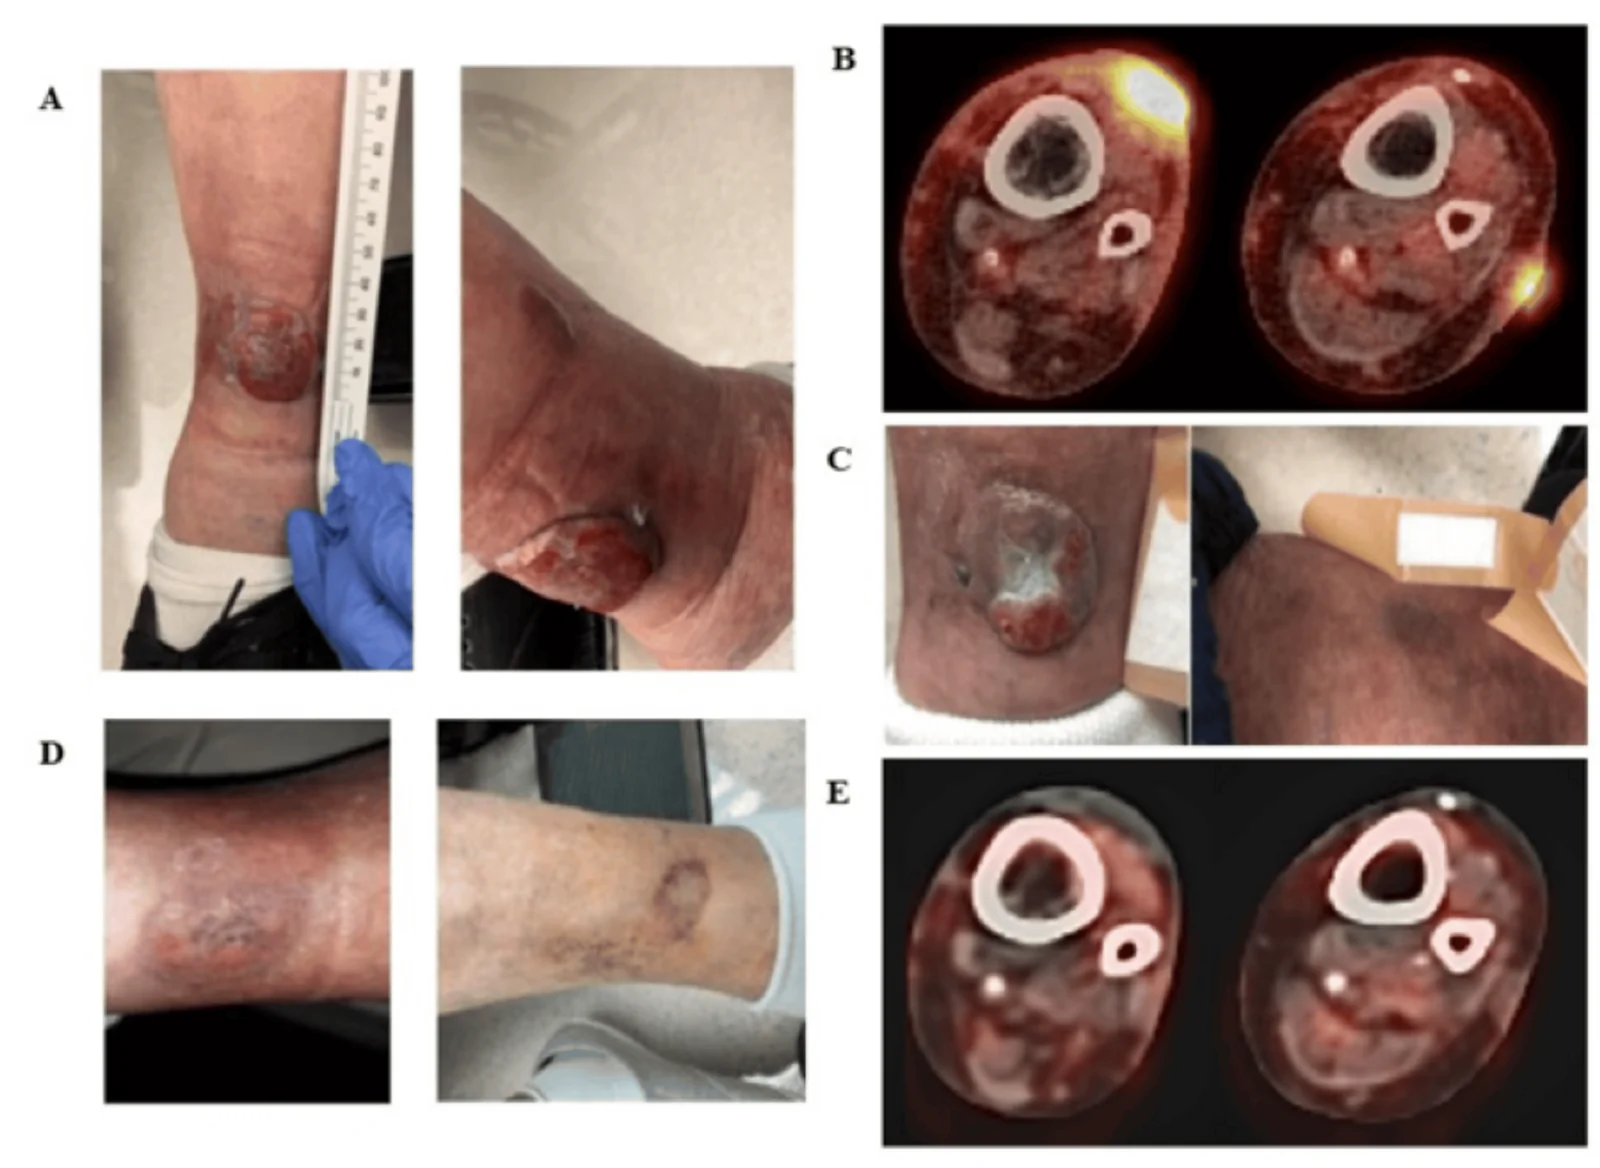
Clinical and imaging progression of the patient’s leg lesion prior to and after radiation monotherapy. (A) The left anterior (left) and left lateral (right) views of the patient’s lesion on her ankle, including the lesion measurement (3.5 cm) in the anterior view. (B) The PET/CT image obtained at diagnosis shows two hypermetabolic cutaneous/subcutaneous lesions on the left distal leg. (C) The lesion prior to initiating radiation therapy. (D) The lesion at seven days (left) and six months (right) after radiation therapy. (E) The PET/CT image obtained three months after radiation therapy demonstrates a complete metabolic response. cm: centimeter; PET/CT: positron emission tomography/computed tomography
